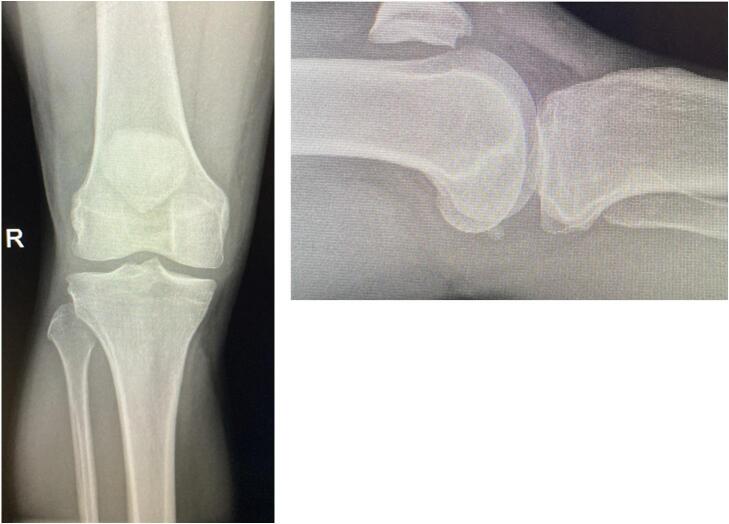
AP and lateral radiograph of right knee.
